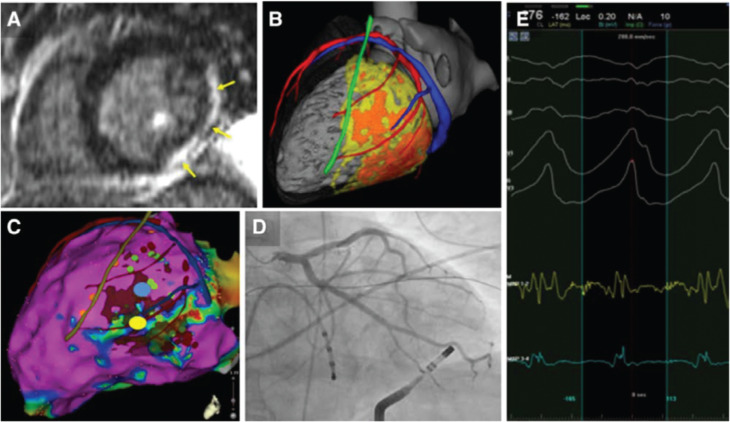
A: Lateral and inferior LV scar on CMR (arrows). B: Patient-specific 3D model built from merged computed tomography (CT) (anatomy) and magnetic resonance imaging (scar) data. Cardiac chambers (gray), coronary arteries, veins (in red and blue, respectively), and left phrenic nerve (green) as segmented from CT and dense scar and gray zone (in orange and yellow, respectively) as segmented from magnetic resonance imaging. C: Epicardial bipolar voltage map with merged imaging model. Voltage mapping (color-coded from purple to red) underestimates the substrate extent as compared with imaging. Fractionated and LPs (green dots) are identified in normal voltage areas. Middiastolic potentials (yellow and blue signals in E) are recorded during VT on an epicardial lateral LV site (yellow dot in C). This potential target for epicardial ablation is far enough from the left phrenic nerve path derived from imaging (green line in C), which accurately matches sites of phrenic capture (orange dots in C). However, CT demonstrates the proximity of this site to a marginal branch of the circumflex artery on the registered imaging model. D: Confirmatory coronary angiography demonstrates contact between the tip of the ablation catheter and the coronary artery. Ablation was thus performed on a different site of the VT isthmus (blue dot in C), resulting in successful VT termination. Reproduced with permission from Mahida S, Sacher F, Dubois R, et al. Cardiac imaging in patients with ventricular tachycardia. *Circulation*. 2017;136(25):2491–2507.
